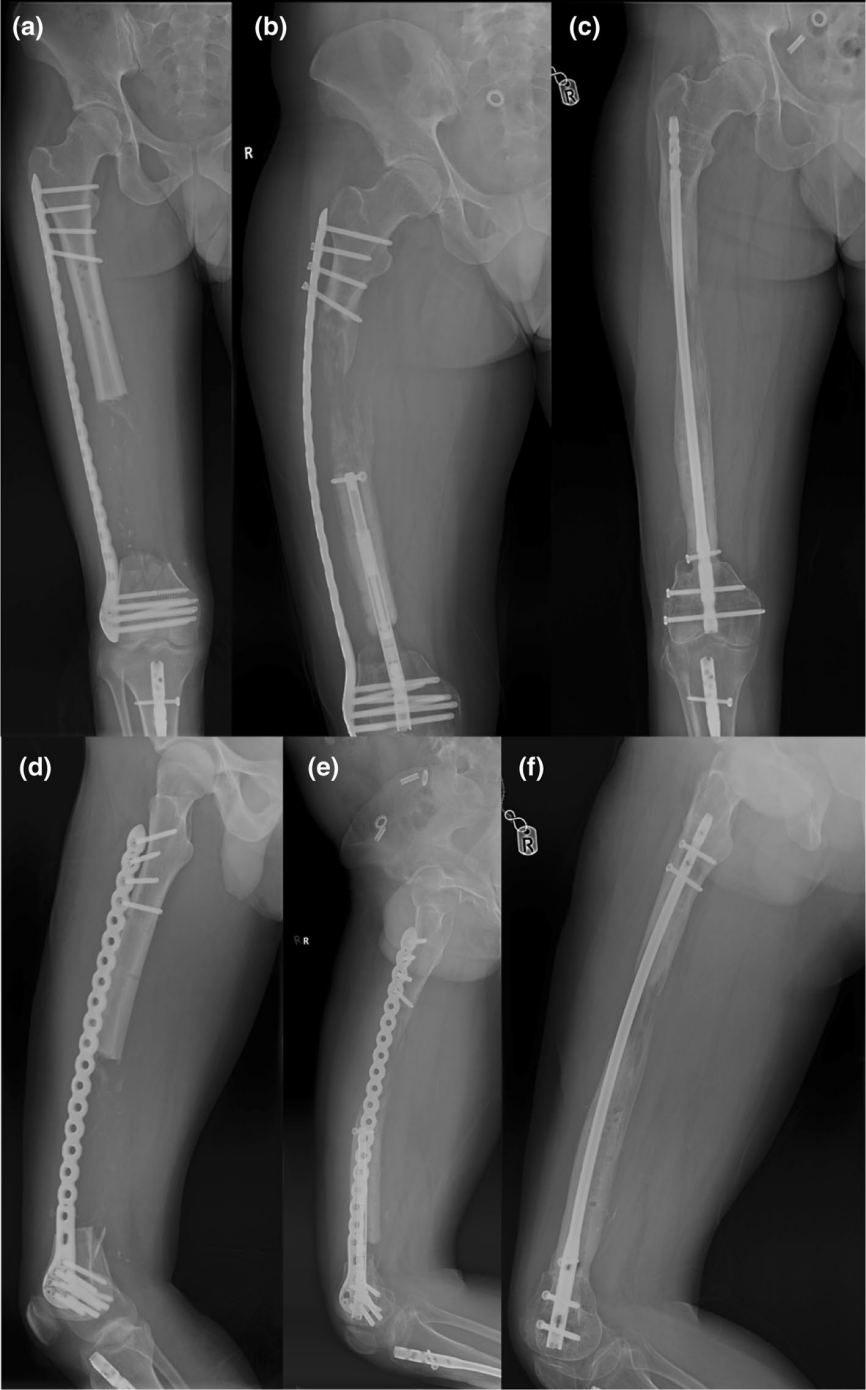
AP (a, b, c) and lateral (d, e, f) radiographs of Patient 3 showing the defect at the start, during the retrograde bone transport and at the final stage. This demonstrates plate bending, as seen in two of our patients
